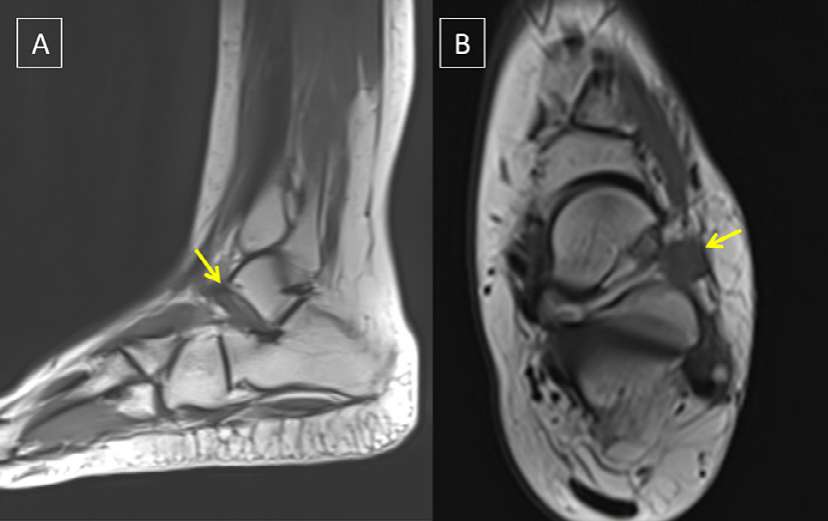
plain MRI, (A) sagittal, (B) axial showing mildly hypointense cystic collection at the dorsolateral aspect of the ankle region.
